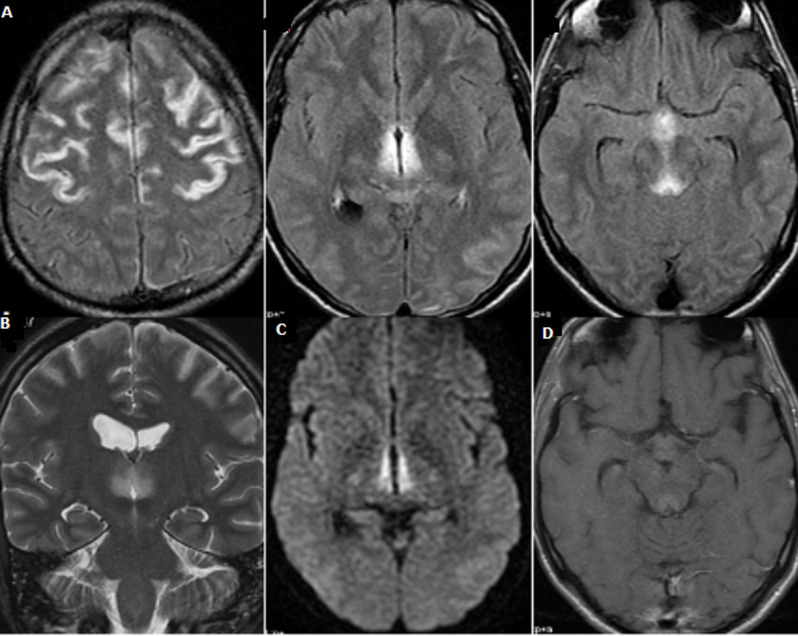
IRM cérébrale en coupe axiale FLAIR (A) et coronale T2 (B) montrant un hypersignal cortical frontal bilatéral symétrique, périaqueducal, des thalami. Il y a une restriction de la diffusion (C) des lésions thalami et discrète rehaussement périaqueducal (D)
